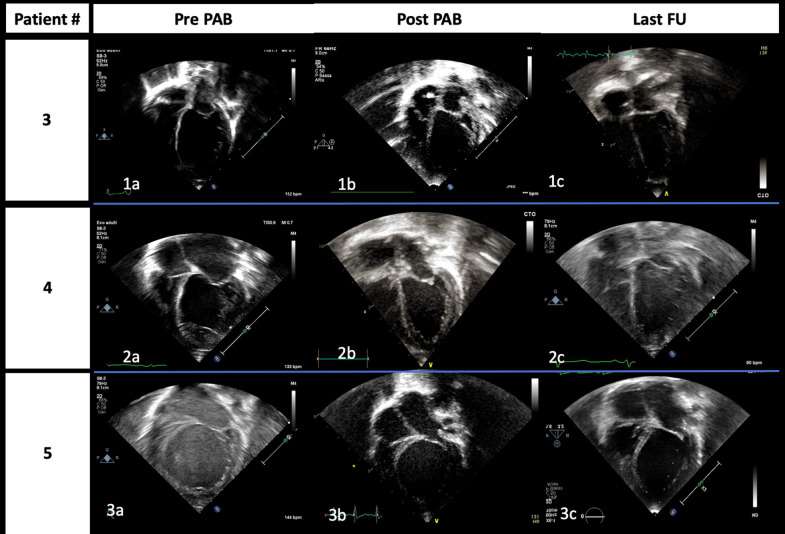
End-diastolic images from apical four-chamber view of patients #3 (1), #4 (2), and #5 (3) acquired at admission (a), immediately after PAB procedure (b), and at the last follow-up (c), respectively. The gradual improvement of left atrium and ventricle dilation is highlighted; it is of note that it is already evident immediately after PAB.
